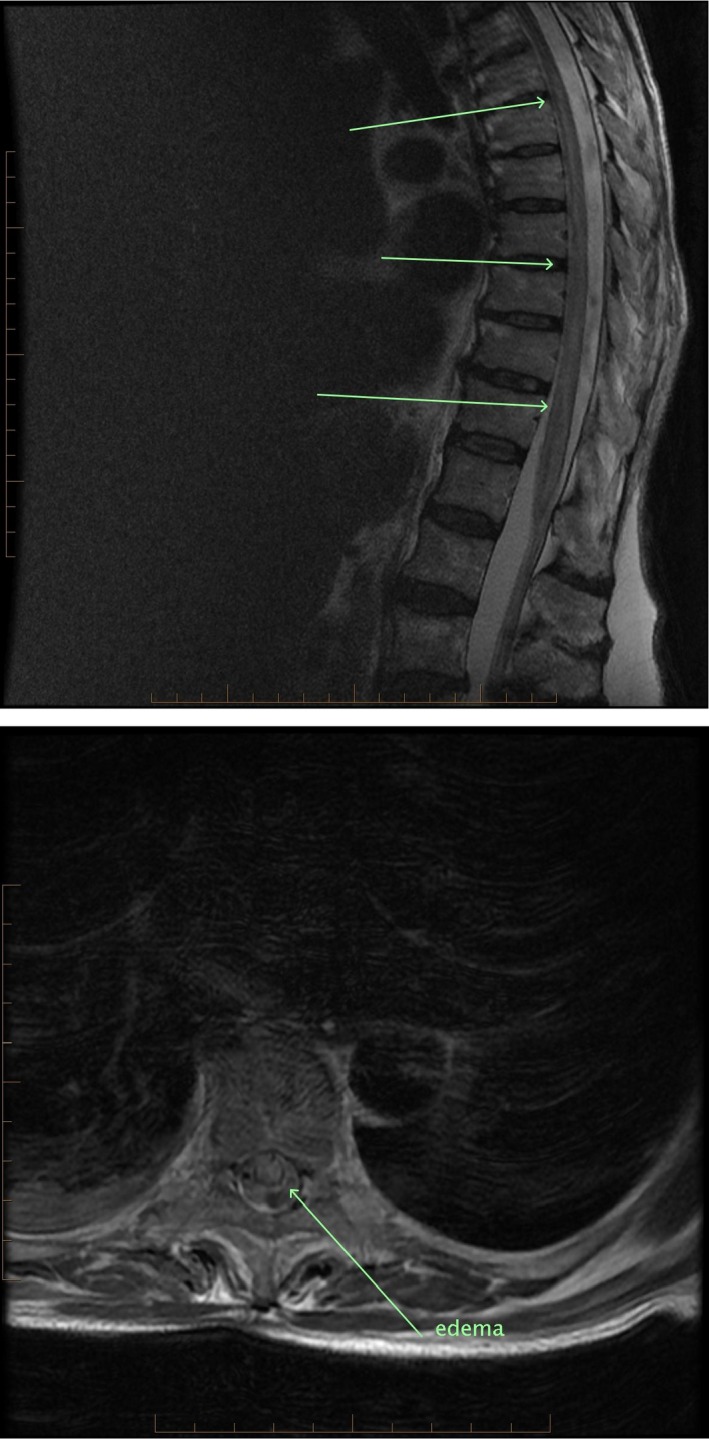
MRI spine showing the extent of involvement from T 6–7 level to conus (image above) and central involvement with thin rim of peripheral sparing of the spinal cord consistent with ASA syndrome.
